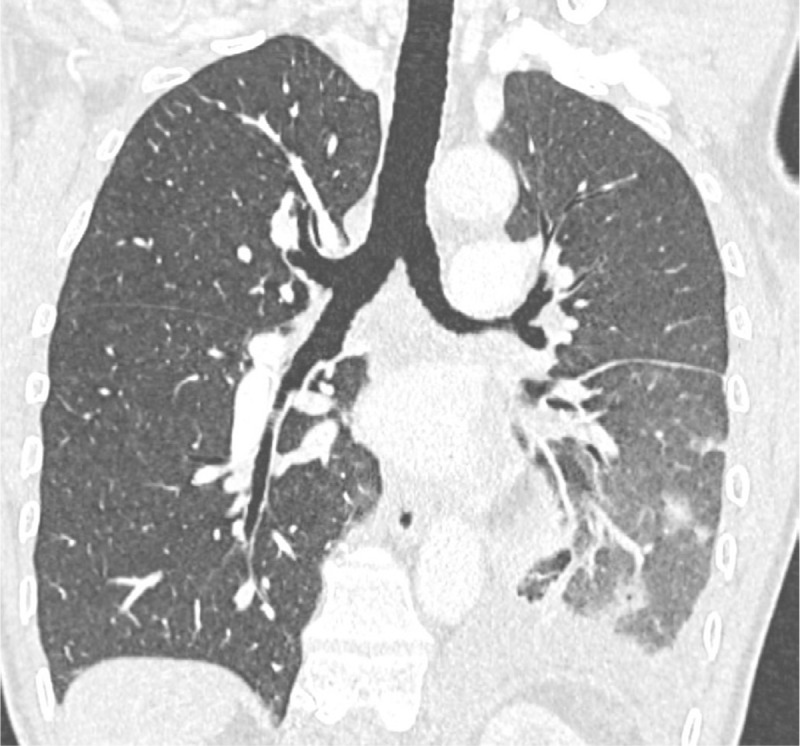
Contrast‑enhanced coronal chest CT (pulmonary window) with unilateral left signs of patchy alveolar hemorrhages, pulmonary edema, and pleural effusion.
